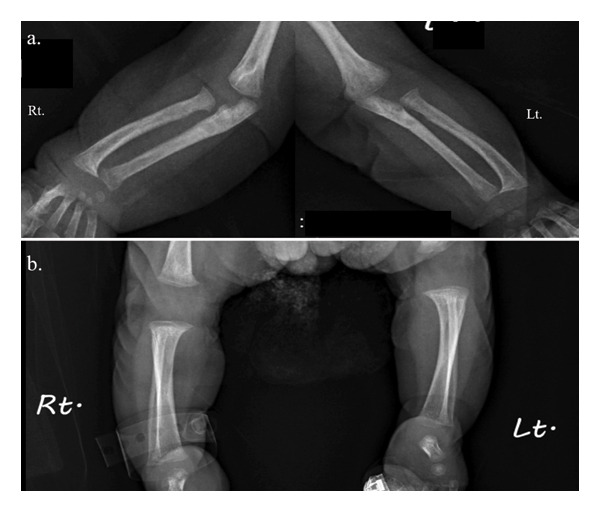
Skeletal radiography of the patient’s long bone (AP). (a) Forearms showed widening of the metaphysis and mild bowing of bilateral radius and ulna. (b) Legs showed widening of the metaphysis and mild bowing of bilateral tibia, overgrowth of bilateral proximal fibula.
